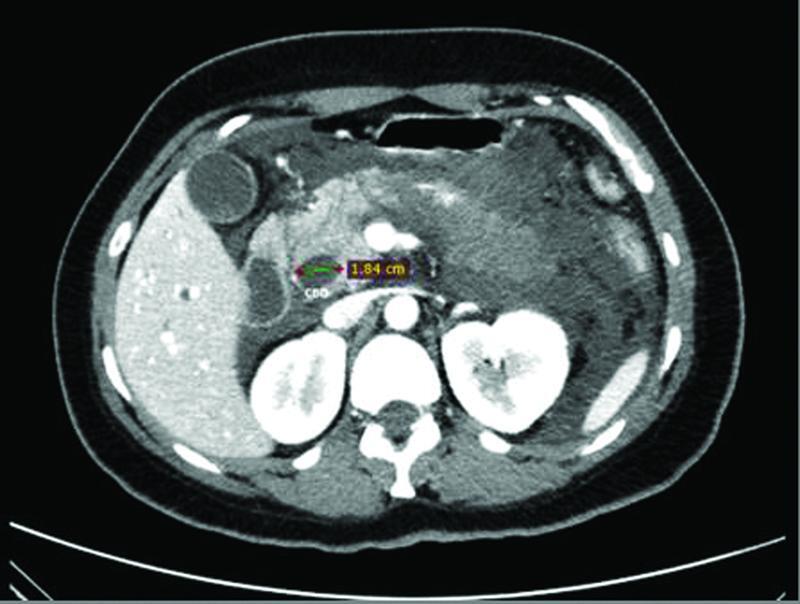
Computed tomography scan image showing the dilated common bile duct (common bile duct diameter: 1.84cm).
